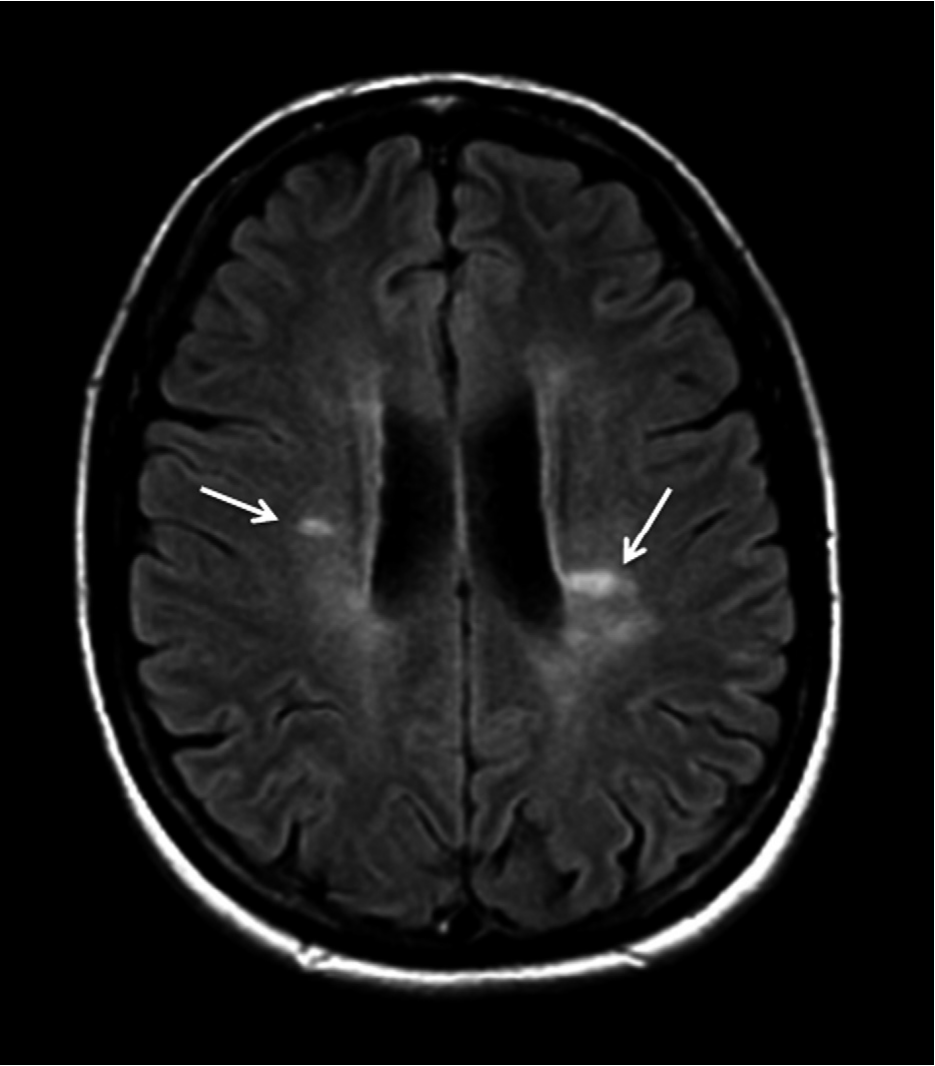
Brain MRI axial fluid attenuated inversion recovery (FLAIR) image shows the characteristic periventricular areas of increased signal intensity (arrows) that are oriented perpendicular to and often contiguous with the lateral ventricles.
